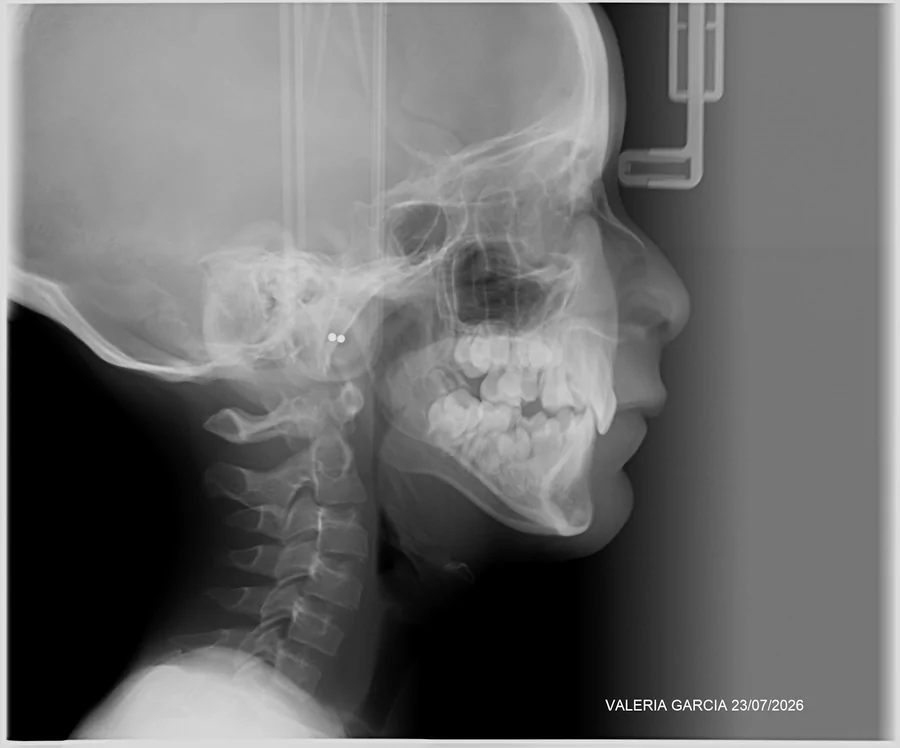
Lateral cephalometric radiograph demonstrating a skeletal class II relationship associated with mild mandibular retrusion and a hyperdivergent growth pattern.
